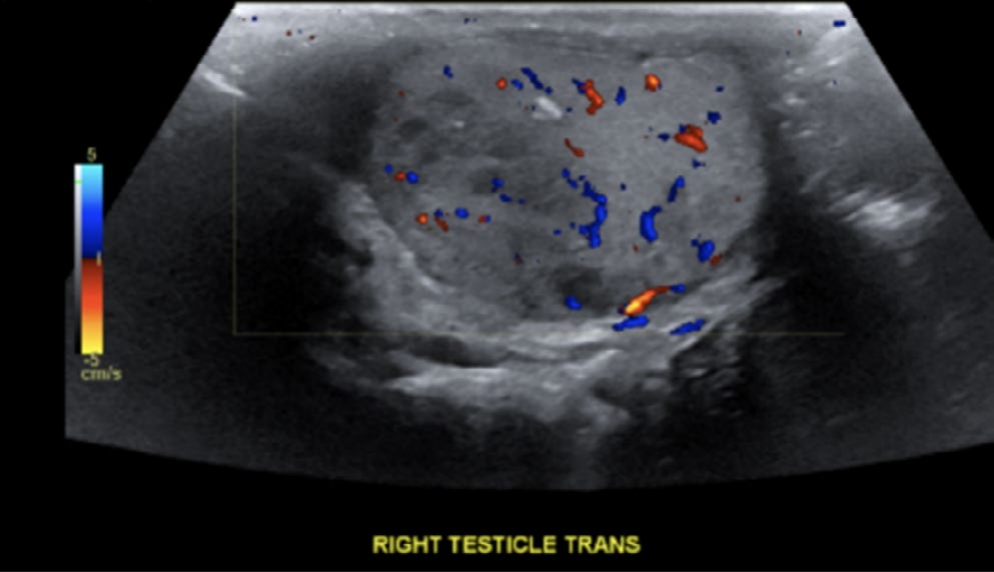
Scrotal ultrasound. Color Doppler and spectral waveform analysis utilized. Heterogeneous right testicular mass measuring 4.2 cm.
